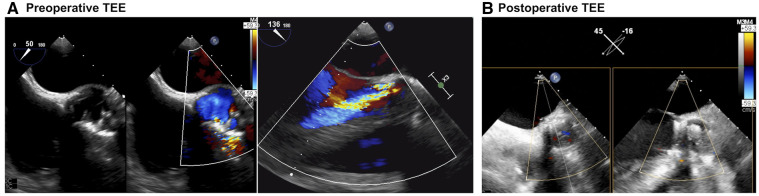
Preoperative and postoperative echocardiogram. (A) Preoperative transesophageal echocardiography (TEE) showed severe paravalvular leakage (PVL) from some aortic annular sutures at the right sinus of Valsalva. (B) No significant PVL was observed postoperatively. Each echocardiogram is shown as a Video 1.
